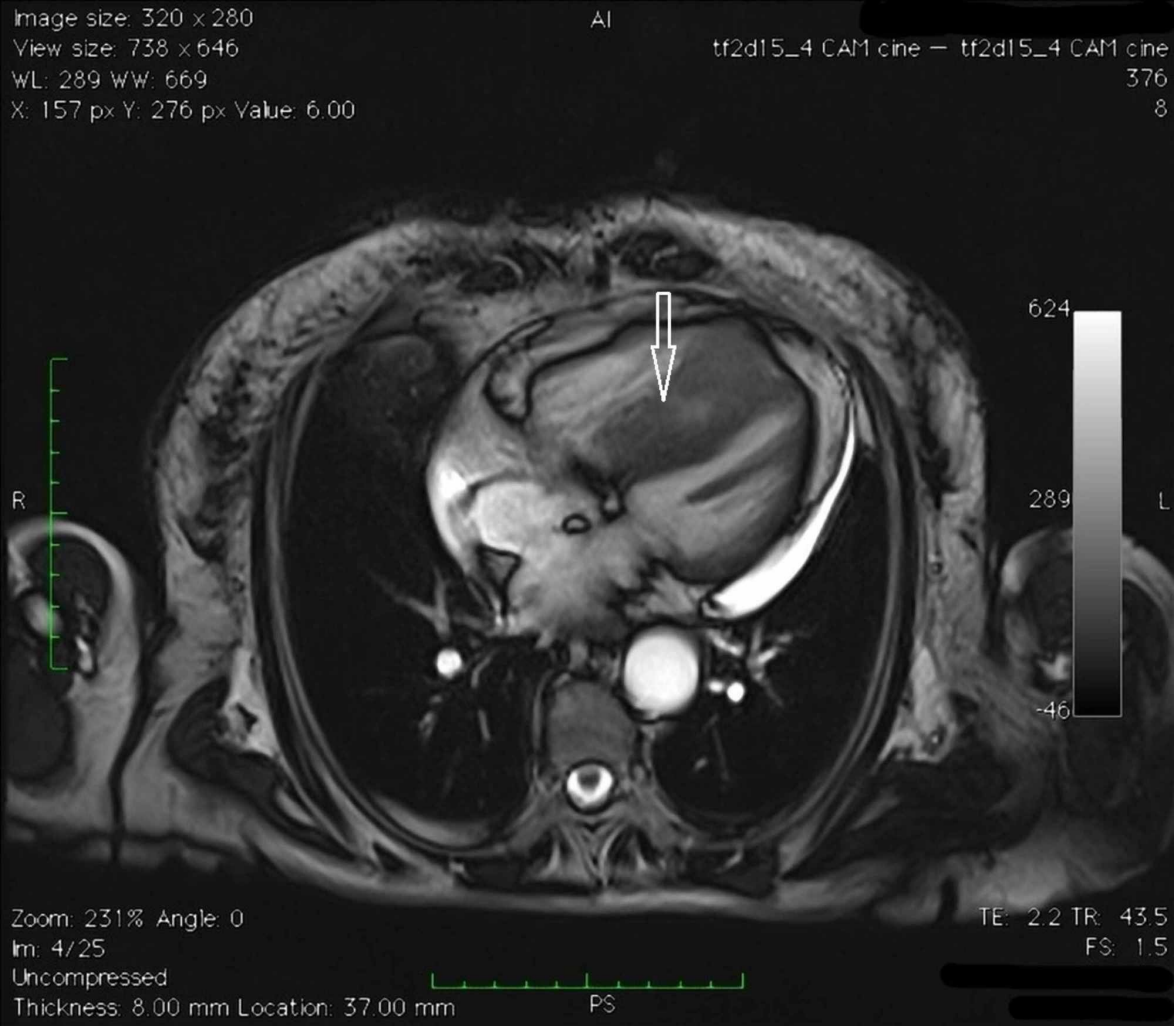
Cardiac magnetic resonance imaging (MRI) Four chambers cine MRI.
